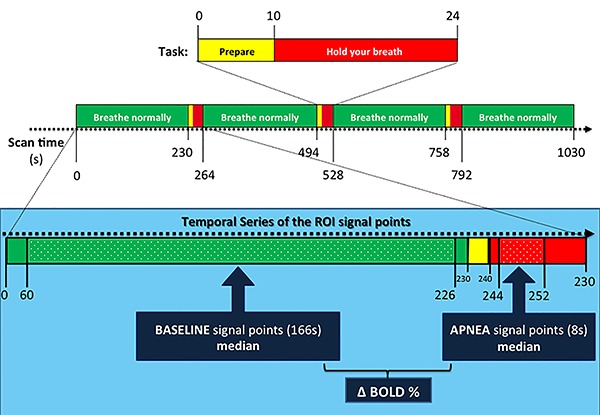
Top*: visual BOLD-functional MRI activation paradigm based on traffic lights for instructing subjects on breathing tasks during echo-planar image acquisition. *Bottom*, within the light blue rectangle: a detailed view of the temporal series of the region of interest (ROI) signal points that were considered for baseline and apnea signal points median calculation, as well as the for the percent variation in the magnitude of stimulus-induced BOLD signal (delta BOLD%).
